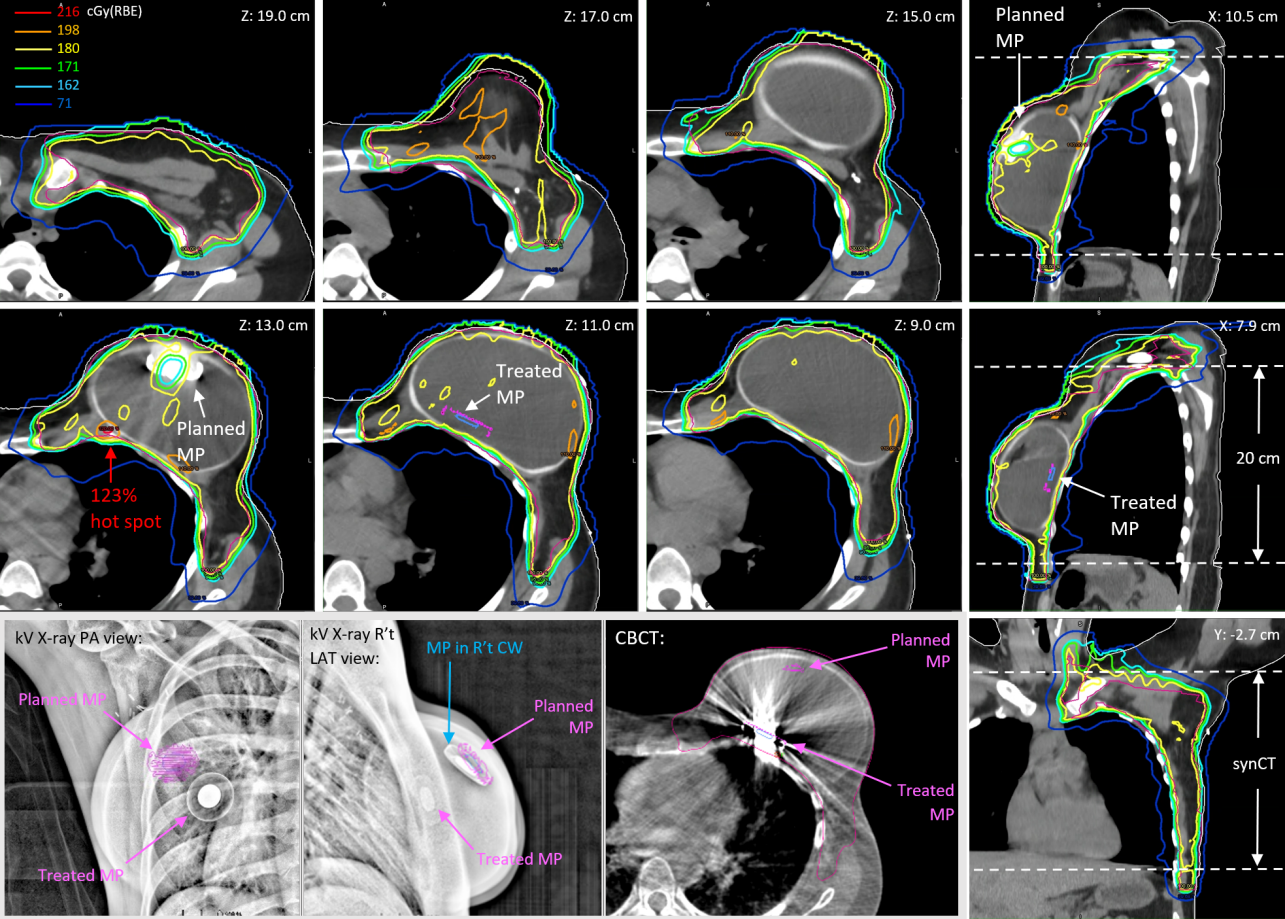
The reconstructed fractional doses on the day of 19 July 2021 for a prescription of 180 cGy(RBE) per fraction. The kV X-ray images and CBCT show the dislocation of the treated MP versus the planned MP.
